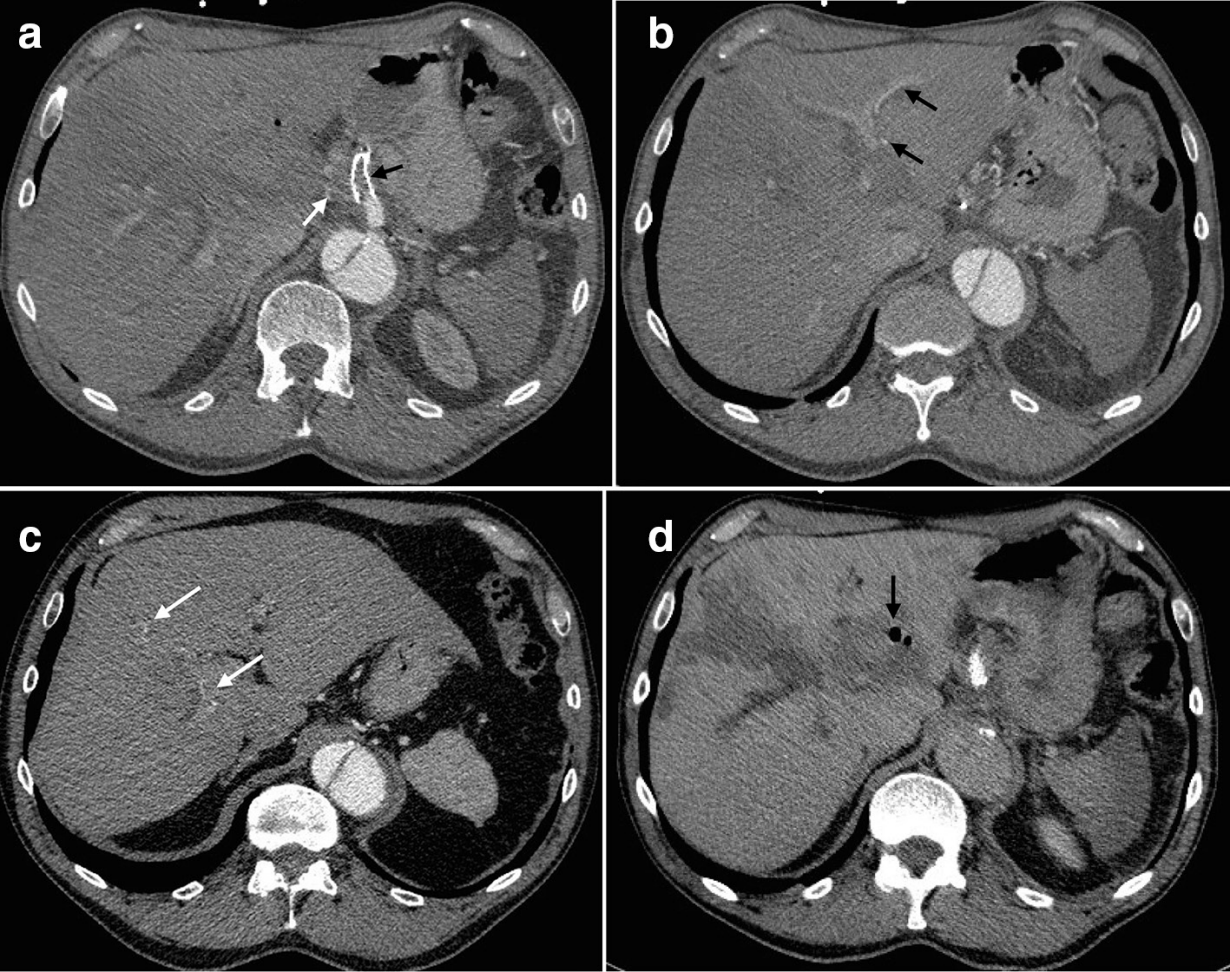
CT performed 3 days post-operatively. (a) Arterial phase imaging shows the stent–graft (black arrow) and common hepatic artery (white arrow) are occluded; Intra hepatic arterial branches filling via collaterals in the left lobe (b, black arrows) and right lobe (c, white arrows - please note these white arrows have moved since the figure has been reformatted); (d) Venous phase imaging showing segment IV hepatic infarction, hypoperfusion in the periphery of the spleenand gas in the excluded aneurysm (black arrow).
